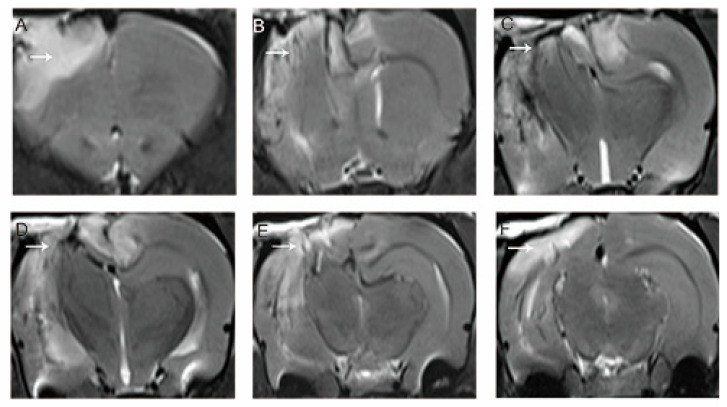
T2-weighted imaging of the brain 1 day after right motor cortex resection. (A–F) Lesions on different sections from anterior to posterior (white arrows). Edema appeared at the resection site and extended to subcortical areas, including the hippocampus and thalamus. In contrast, the structure of the contralateral hemisphere was intact; however, a high T2 signal representing hemorrhage was obvious around the midline.
